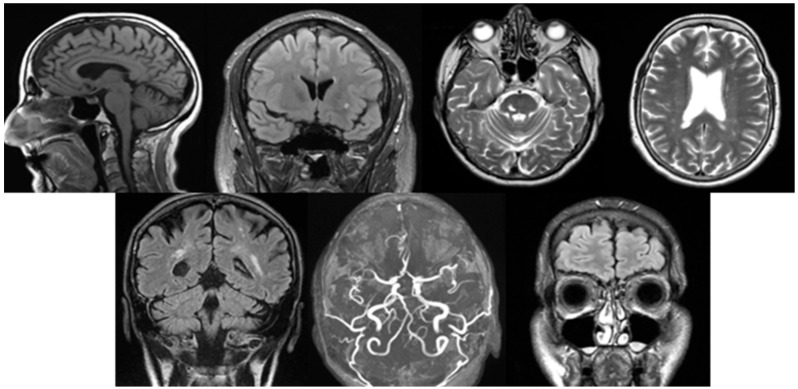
From left to right in the first row are cortical atrophy (sagittal T1-weighted, cortical thickness and widening of the sulci), white matter lesion (coronal FLAIR, hyperintense periventricular changes), vascular lesions (axial T2-weighted, focal hyperintensity), and lacunar lesion (axial T2-weighted, hyperintense deep white matter changes), and in the second row are vascular encephalopathy (coronal FLAIR, diffuse white matter hyperintensities), circle of Willis variation (axial TOF-MRA, anatomical variation in the arterial configuration), sinusitis (coronal FLAIR, mucosal thickening and sinus opacification).
